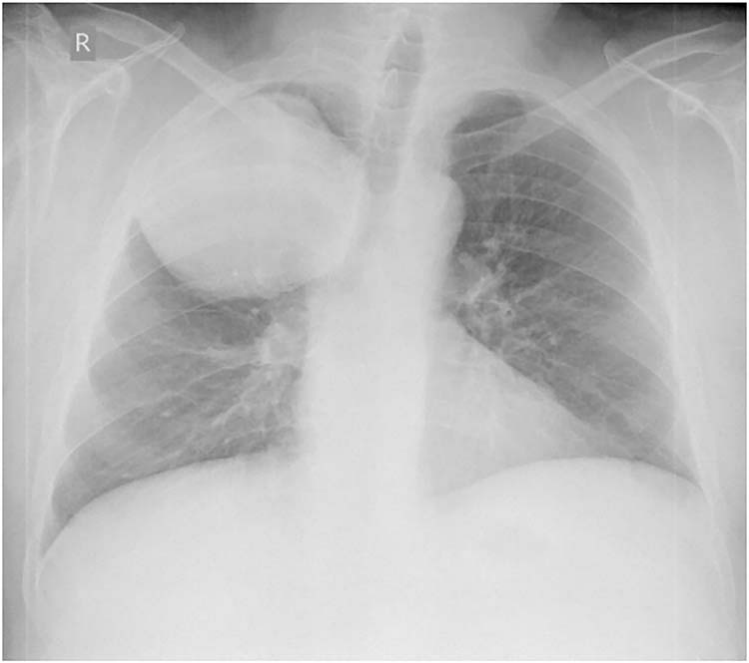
Anteroposterior chest X-ray. Giant, homogeneous, clearly circumscribed opacity in the upper part of the right chest.
